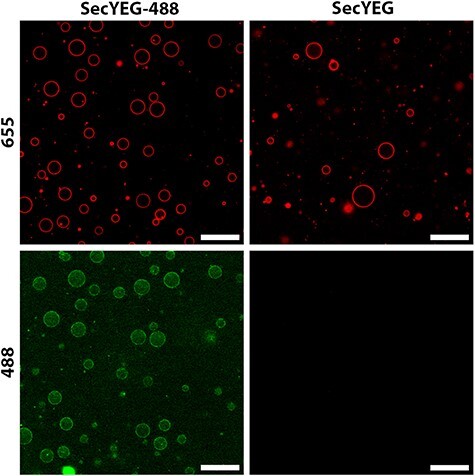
Formation of SecGUVs from SecSUVs containing SecYEG-AF488. Differences in GUV quantity are due to random variation within the field of view. Lipid composition DOPC:DOPE:DOPG 40:30:30 supplemented with 0.1 mol% DOPE-ATTO655. Red 655 channel: DOPE-ATTO655. Green 488 channel: SecYEG-488. Scale bars: 50 µm.
